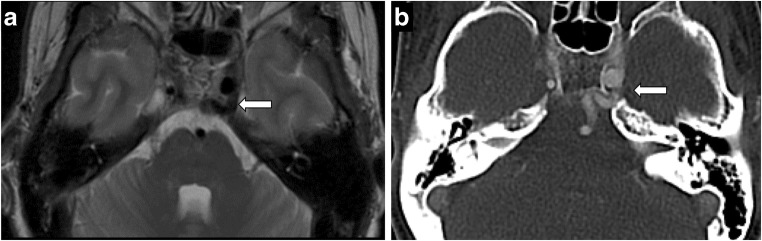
Persistent trigeminal artery. Axial T2-weighted imaging (a) and CT angiography (b). Vascular channel connecting the left internal carotid artery and the basilar artery, and running through the medial aspect of Meckel’s cave
